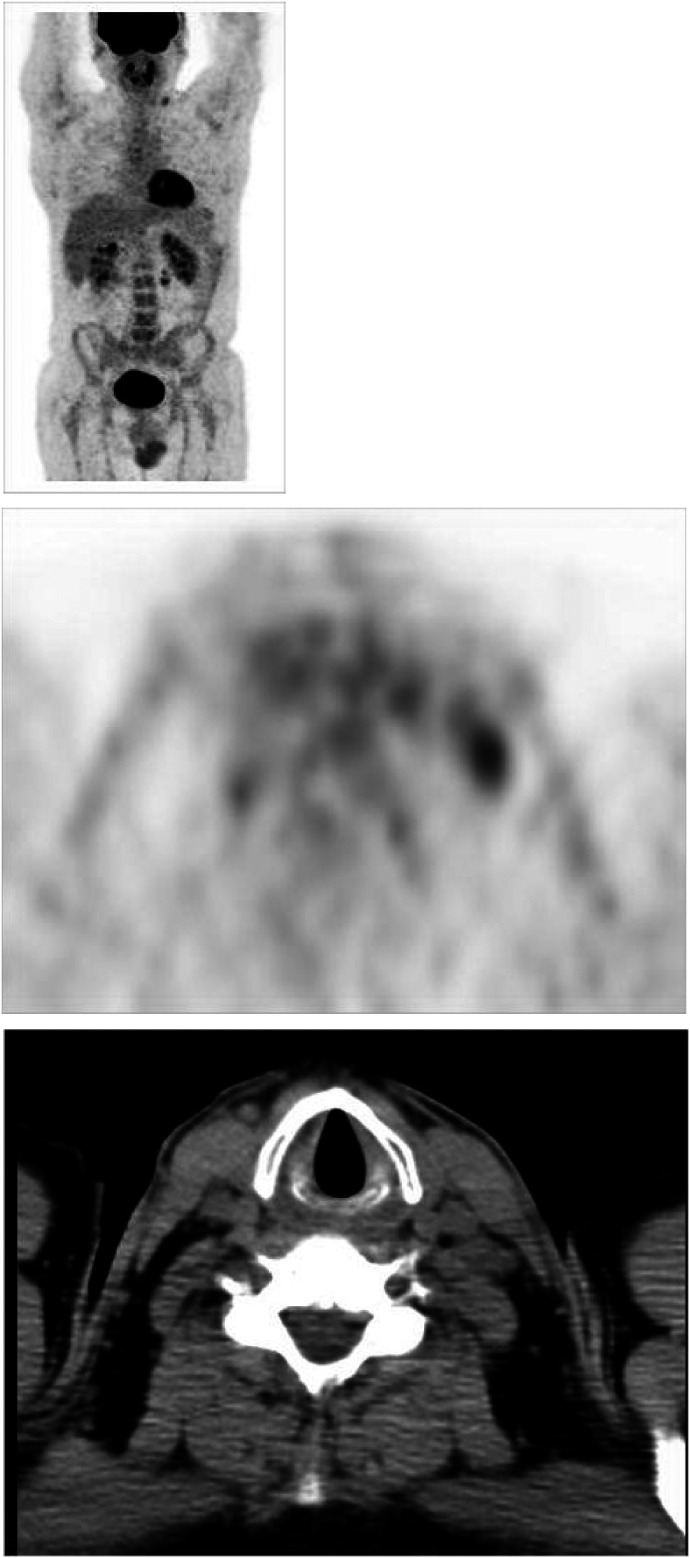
FDG PET (maximum intensity projection – MIP-image) showing an area of increased uptake in a left latero-crevical lymph node and a second area of increased uptake in abdomen, likely consistent with juxtaintestinal lymph node. Corresponding transaxial PET and CT images show the enlarged left laterocervical lymph node. Biopsy was positive for lymphoma relapse.
